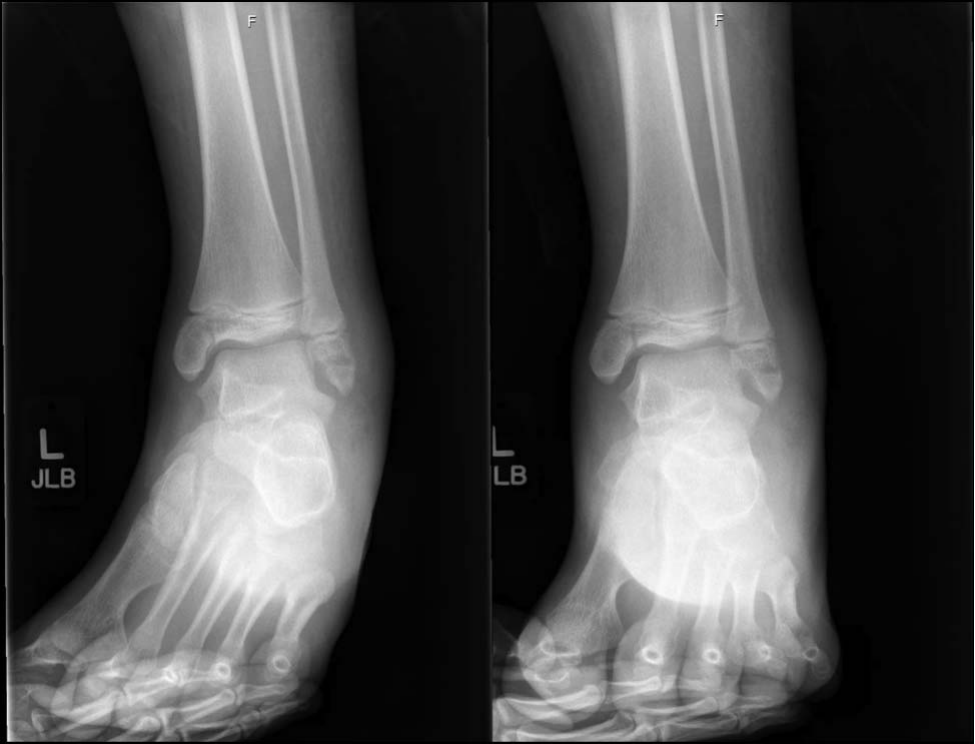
AP and mortise view ankle radiographs of Case 2 after their injury, demonstrating a displaced Salter-Harris type III medial distal tibia fracture and Ogden Type VII distal fibula fracture.
